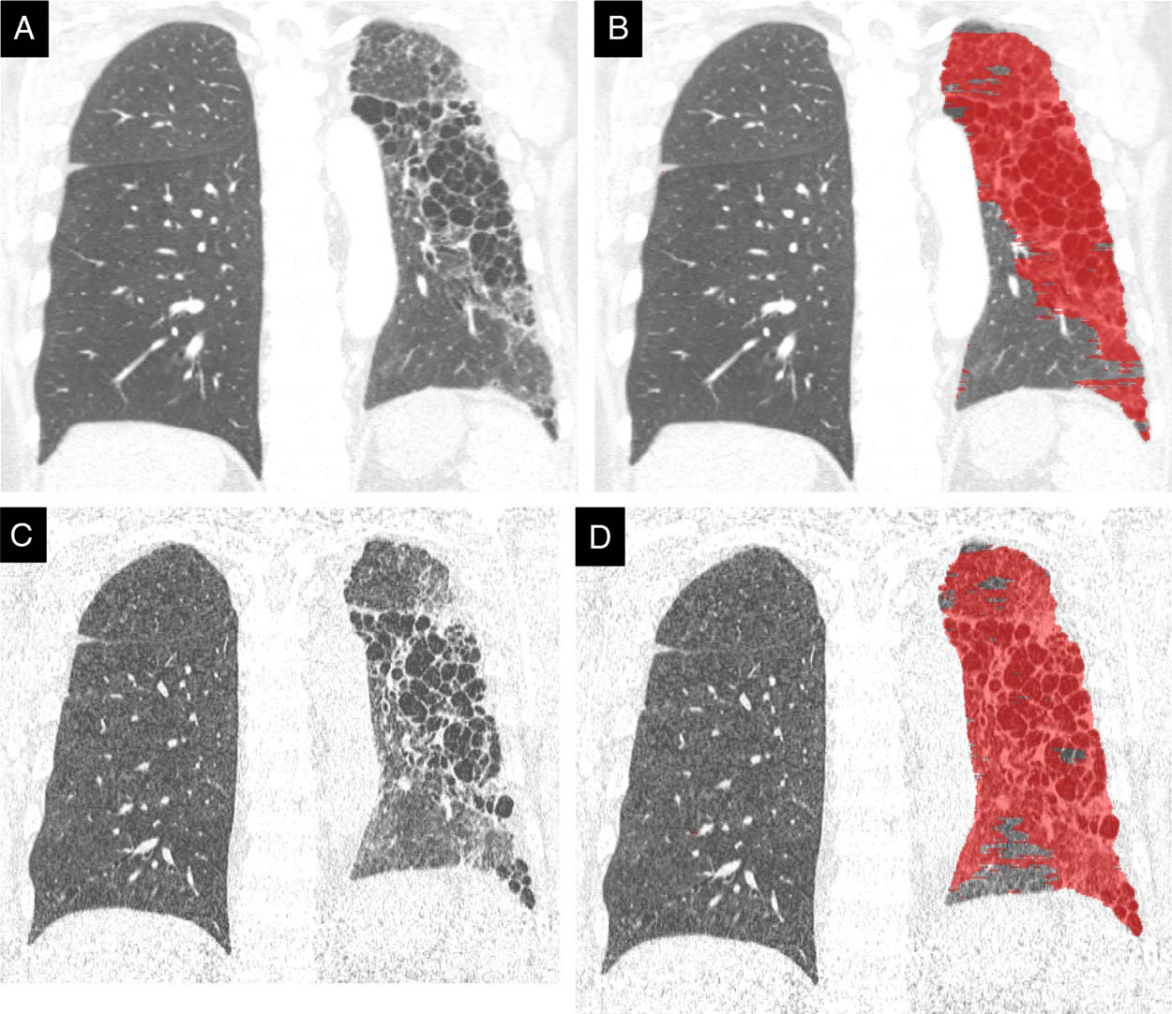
A 42-year-old woman with fibrosing mediastinitis, the same case shown in Figure 2. A and C, High-resolution CT shows fibrosis of the left lung in a UIP pattern at baseline (A) and at 2-year follow-up (C), with corresponding quantification of the fibrotic abnormalities (B and D). Fibrosis has progressed over 2 years, with increased reticulations and cystic abnormalities. DTA quantified a fibrotic score (color overlay) of 57.2% at baseline and 66.6% at follow-up; specifically, the percentage of reticular abnormalities was increased, and the lung volume was further reduced.
